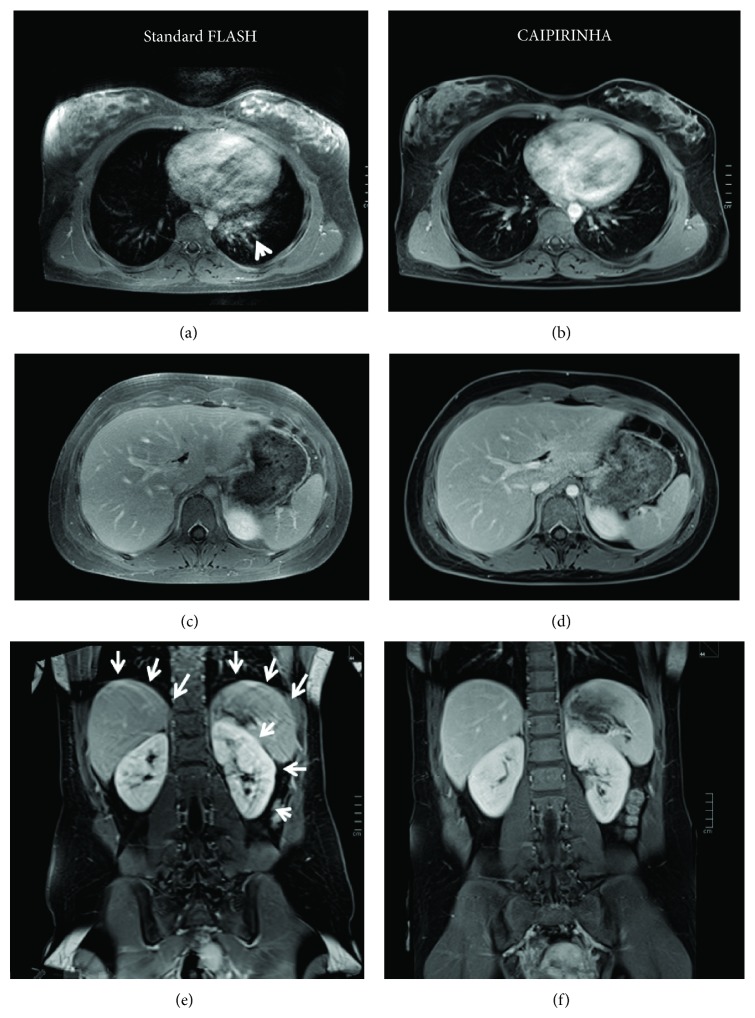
Thoracic and abdominal MRI study in a 17-year-old female patient with Hodgkin's disease in complete remission. Images are displayed for contrast-enhanced fat-saturated standard FLASH (a, c, e) and CAIPIRINHA-accelerated T1w 3D FLASH (b, d, f) from the transverse thoracic image stack (a, b), the transverse abdominal image stack (c, d), and the coronal abdominal image stack (e, f). The sequences were scanned in turns on the respective level as standard FLASH first, followed by CAIPIRINHA. With CAIPIRINHA, there are markedly less retrocardial artefacts ((b), as compared to standard FLASH (a), arrowhead). While the transverse thoracoabdominal scans otherwise show excellent image quality with both FLASH and CAIPIRINHA, the patient could eventually no longer fully comply with the frequent breath-holds, so that the coronal FLASH image (e, arrows) is degraded by respiratory motion artefacts and shows blurring of contours along the diaphragm and the kidneys. The final acquisition of the coronal CAIPIRINHA sequence (f) again is virtually free of artefacts. The transverse abdominal scans were included in the study analysis and were consistently graded as IQ = 5 by both readers.
